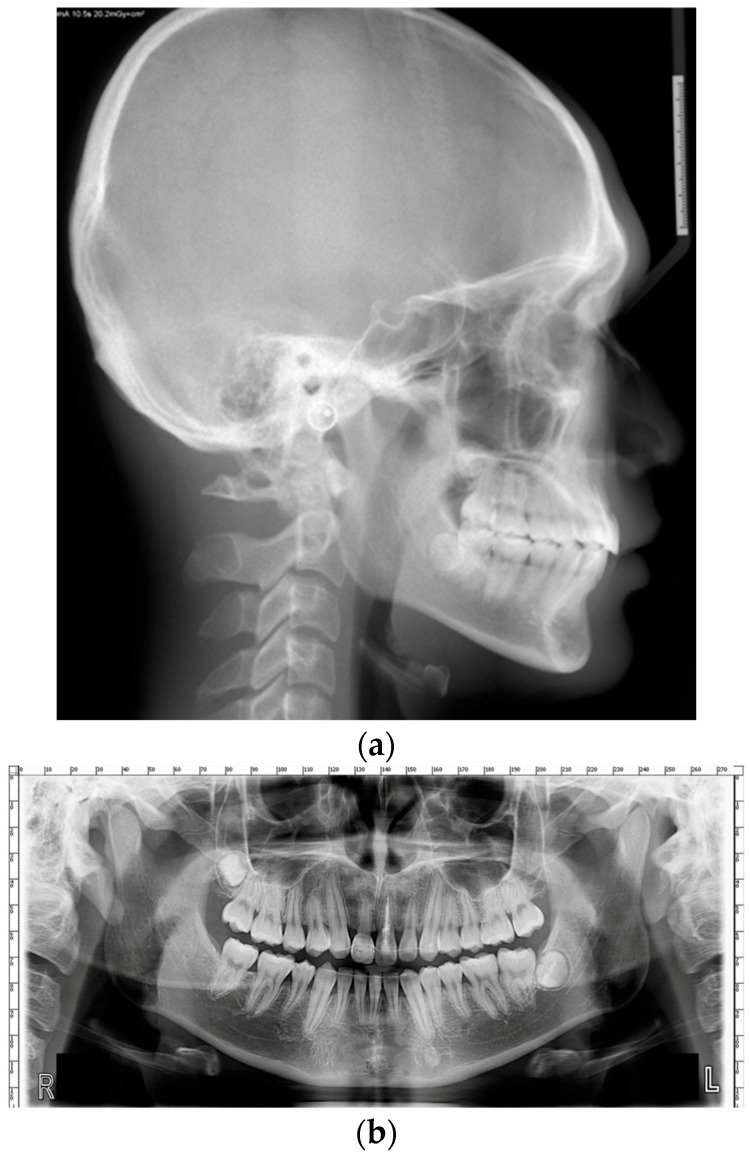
Cephalometric (a) and panoramic (b) X-rays five years after autotransplantation, December 2025.
